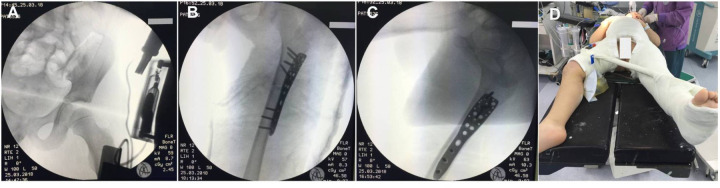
Intraoperative x-rays and postoperative casting for immobilization. (A) Anteroposterior radiograph of the left hip showed a reduced hip joint. (B,C) Intraoperative anteroposterior and lateral x-rays revealing fracture and dislocation reduction and fixation with an adult proximal humerus locking plate and screws. (D) A one-and-a-half-leg hip spica cast was performed immediately after surgery.
